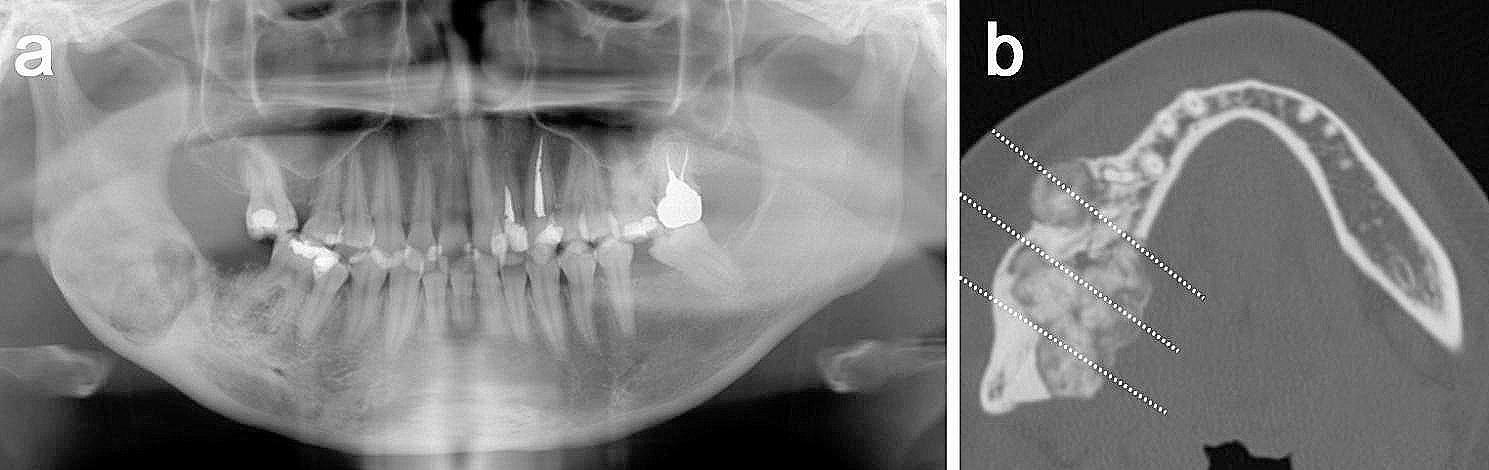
Imaging findings of recurrent lesion. (a) Panoramic radiograph revealed a large, radiopaque lesion in the postoperative region. (b) Axial cone-beam computed tomography image showed multicentric masses surrounded by a thin radiolucent zone. Dotted lines indicate the site of the cut surface, as shown in Fig. 3
